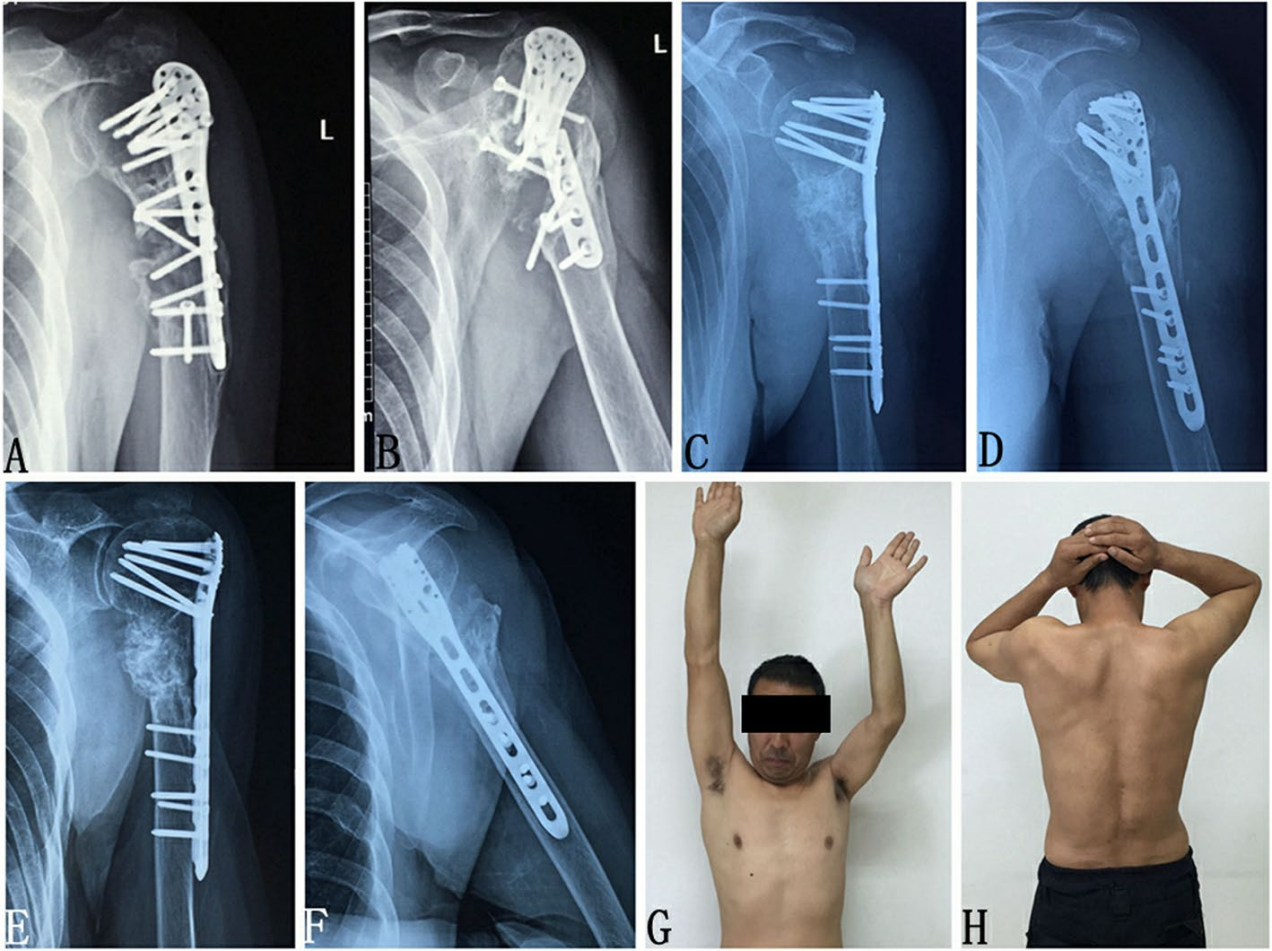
Patient 9, who fell from a height of 4 m 11 years earlier, resulting in ‘left proximal humerus fracture and lumbar fracture’, received surgical treatment in the local hospital. At 8 years ago, the left shoulder had gradually become painful and weak, with limited movement of the left shoulder joint, which was untreated. a, b Preoperative X-ray shows typical synovial pseudoarticular bone nonunion and broken internal fixation; c, d Postoperative X-ray shows that the proximal end of the left humerus is well aligned and the bone graft is sufficient; e, f X-ray at 6 months after the operation shows healed fracture; g, h Although the left shoulder joint is slightly limited in movement and rotation, the patient is satisfied with the treatment results
